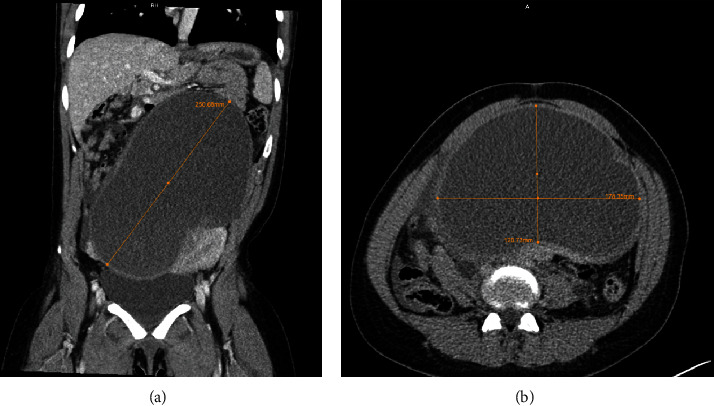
Preoperative contrast-enhanced CT revealed a large unilocular complex adnexal mass in the coronal (a) and axial (b) views, producing a mass effect on the surrounding structures.
